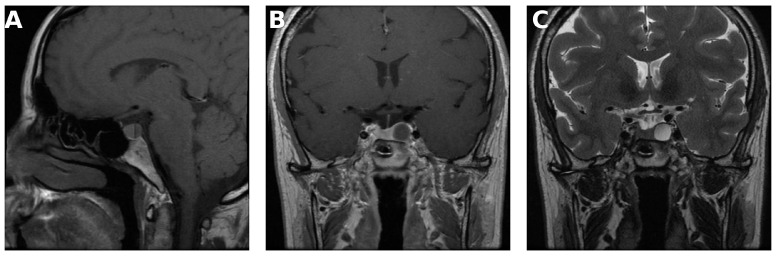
(A) Sagittal T1-weighted image demonstrating a fluid–fluid level within a cystic pituitary adenoma. (B) Contrast-enhanced coronal T1-weighted image demonstrating paramedian localization. (C) Coronal T2-weighted image demonstrating a hypointense rim.
